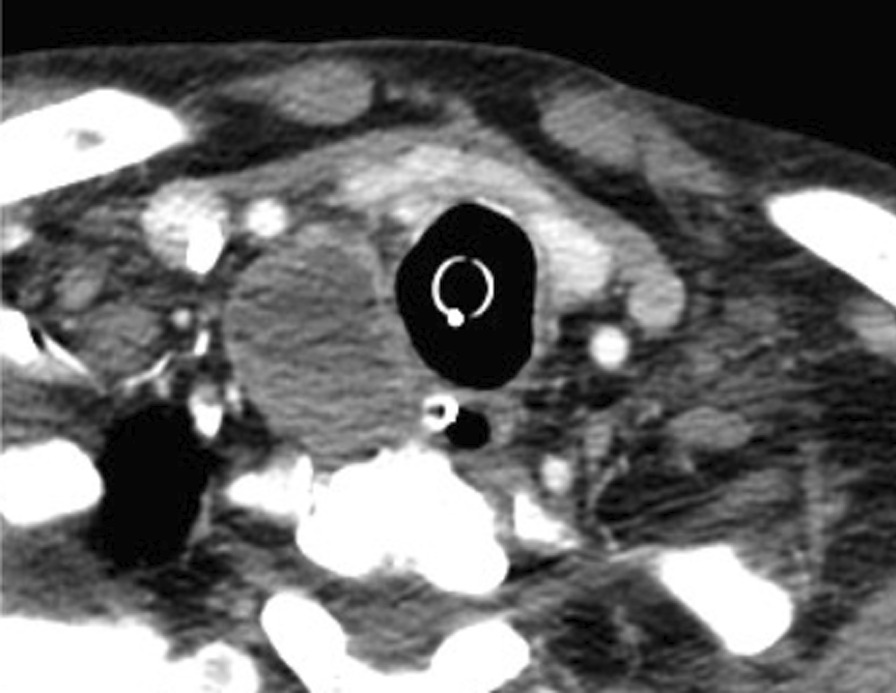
CT neck revealing right paratracheal neck mass
